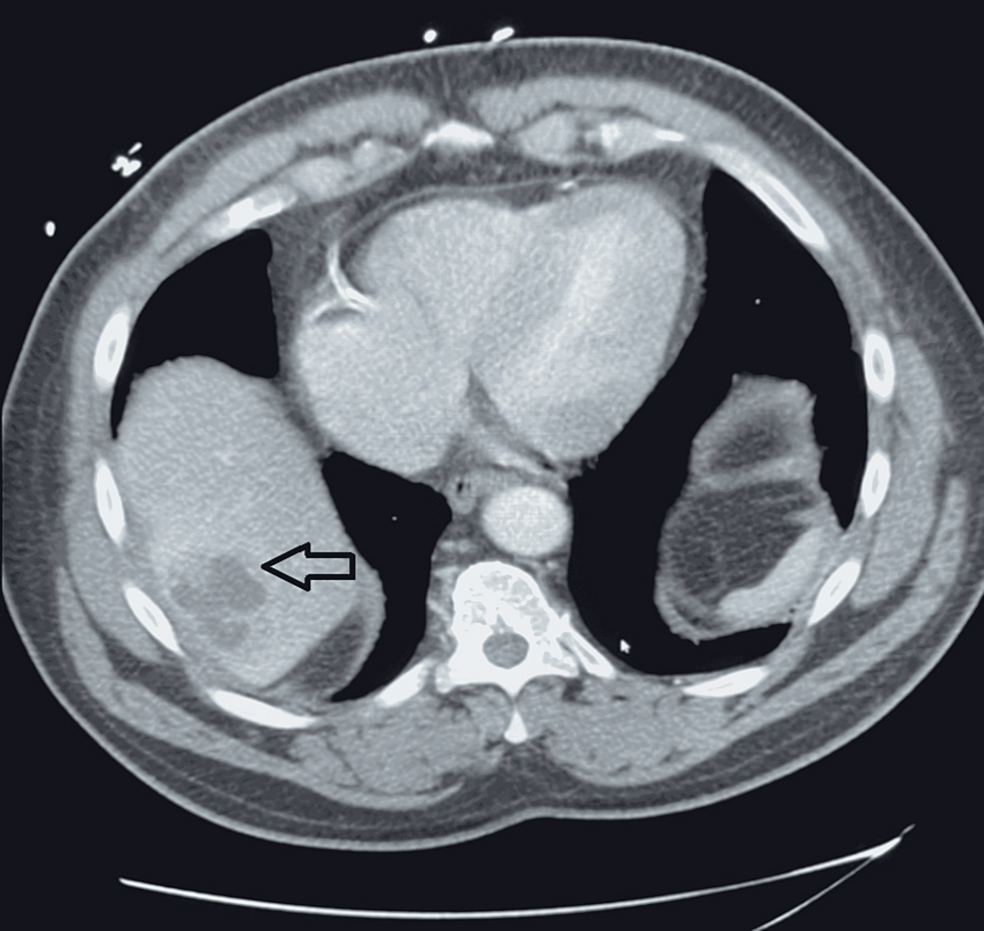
Transverse abdominal computed tomography scan showing a complex cystic area in the right lobe of the liver consistent with an abscess (black arrow)
